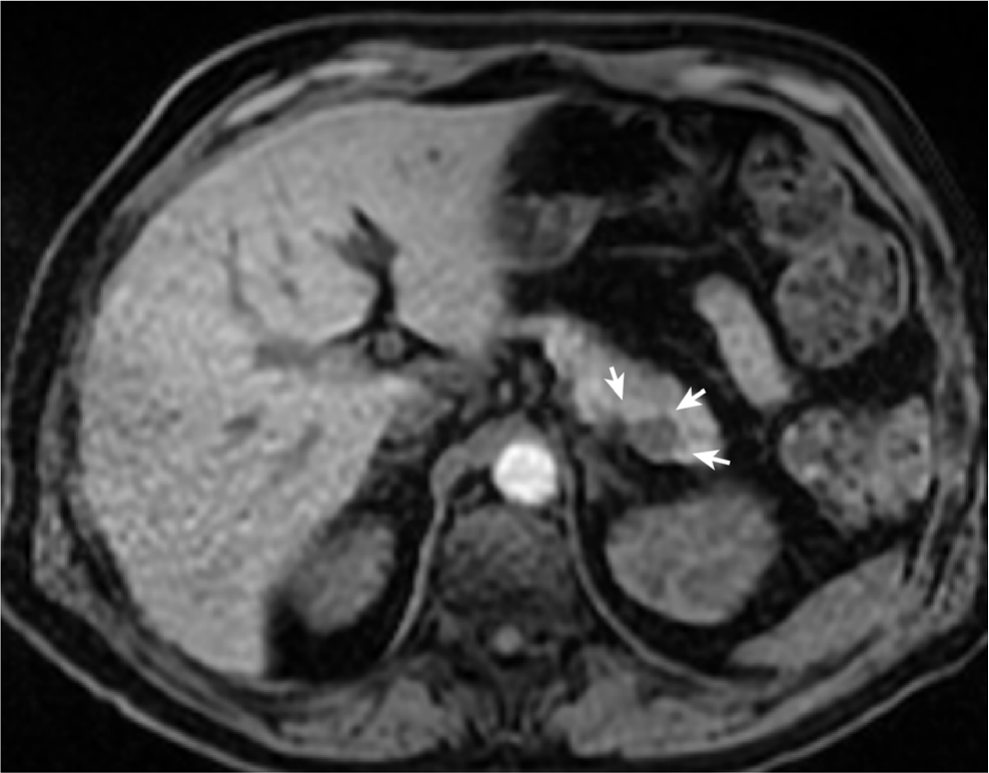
Magnetic resonance imaging (T1 sequence) shows the same lesion identified by CT as a 17 × 14-mm heterogeneously enhanced lesion (*white arrows*) of the pancreatic tail
